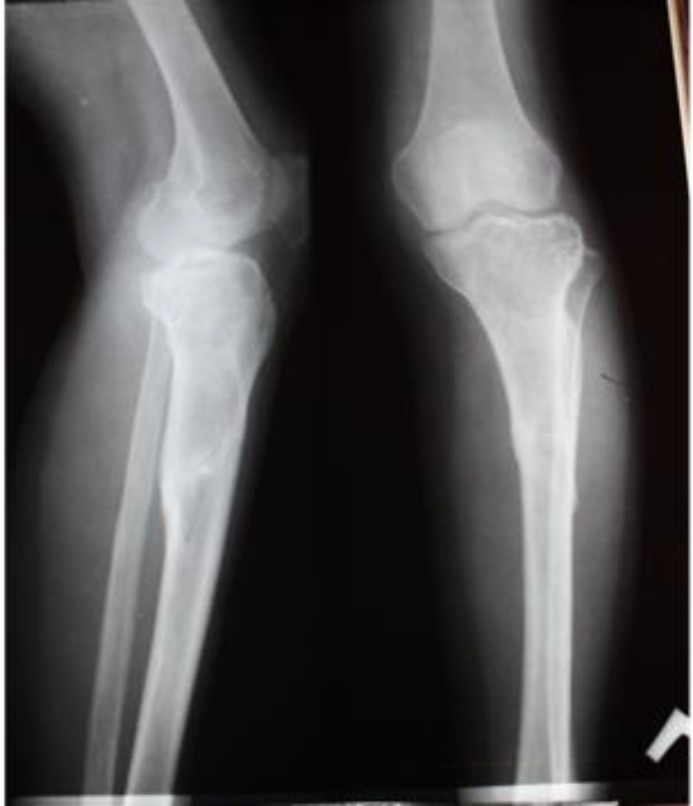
Plain radiograph of a 32-year old female with left knee GCTOB at the first presentation shows lytic expansile well-defined meta-diaphyseal lesion extending to the subarticular region
